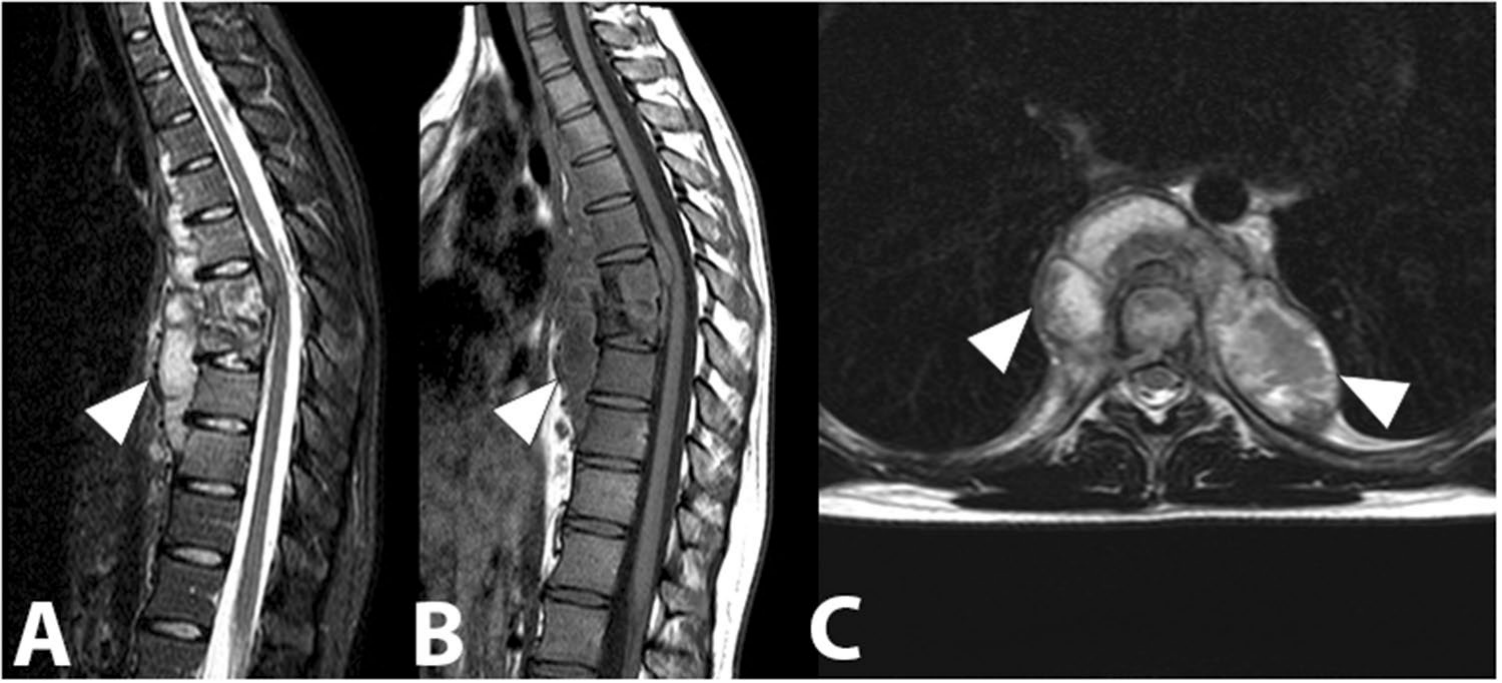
Tubercular spondylodiscitis with altered signal and morphology of vertebral bodies D7 and D8 in sagittal STIR (a) and T1-weighted images (b), absence of intervertebral disc space D7–D8 and presence of paraspinal abscesses extended from D3 to D9, better assessable in axial images (c) (arrowheads)
